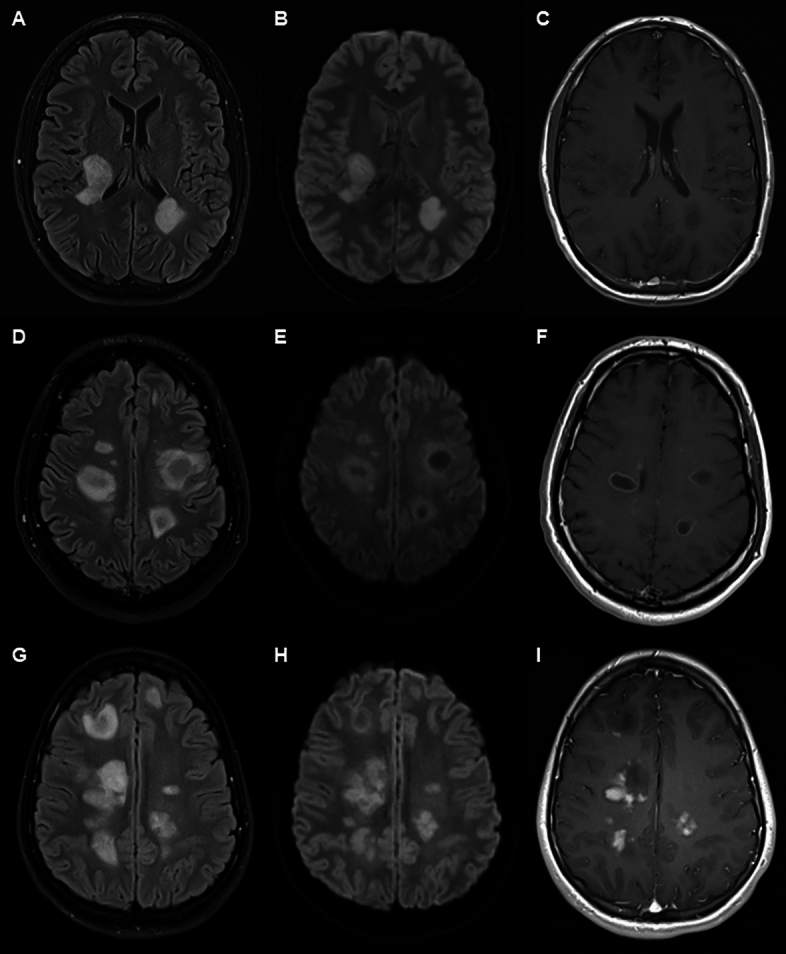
Three patterns of lesion in ADEM-like lesions. Vertical panels show FLAIR sequences (right), diffusion weighted images (centre) and T1 with contrast (left). Horizontal panels show individual cases. Upper panel (A–C) shows case with FLAIR and DWI hyperintensity with T1 hypointensity, but no Gd-enhancement. Middle panel (D–F) shows ring pattern hyperintensity on FLAIR and DWI with central hypointensity and ring-enhancement on T1 sequence. Lower panel (G–I) shows predominantly heterogeneous FLAIR and DWI hyperintensity with heterogeneous Gd-enhancement. There are additional lesions showing central T1 hypointensity and no enhancement.
